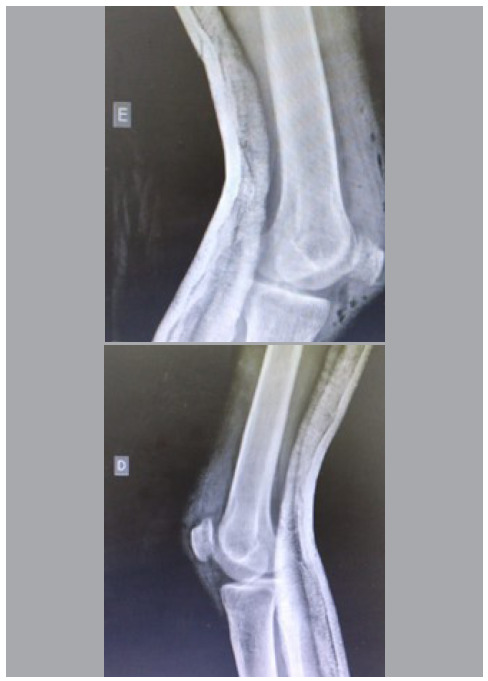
Postoperative radiographs showing the return of patellar height to normal parameters, with a Caton-Deschamps index of 1.0 on the right side and 0.9 on the left side.
